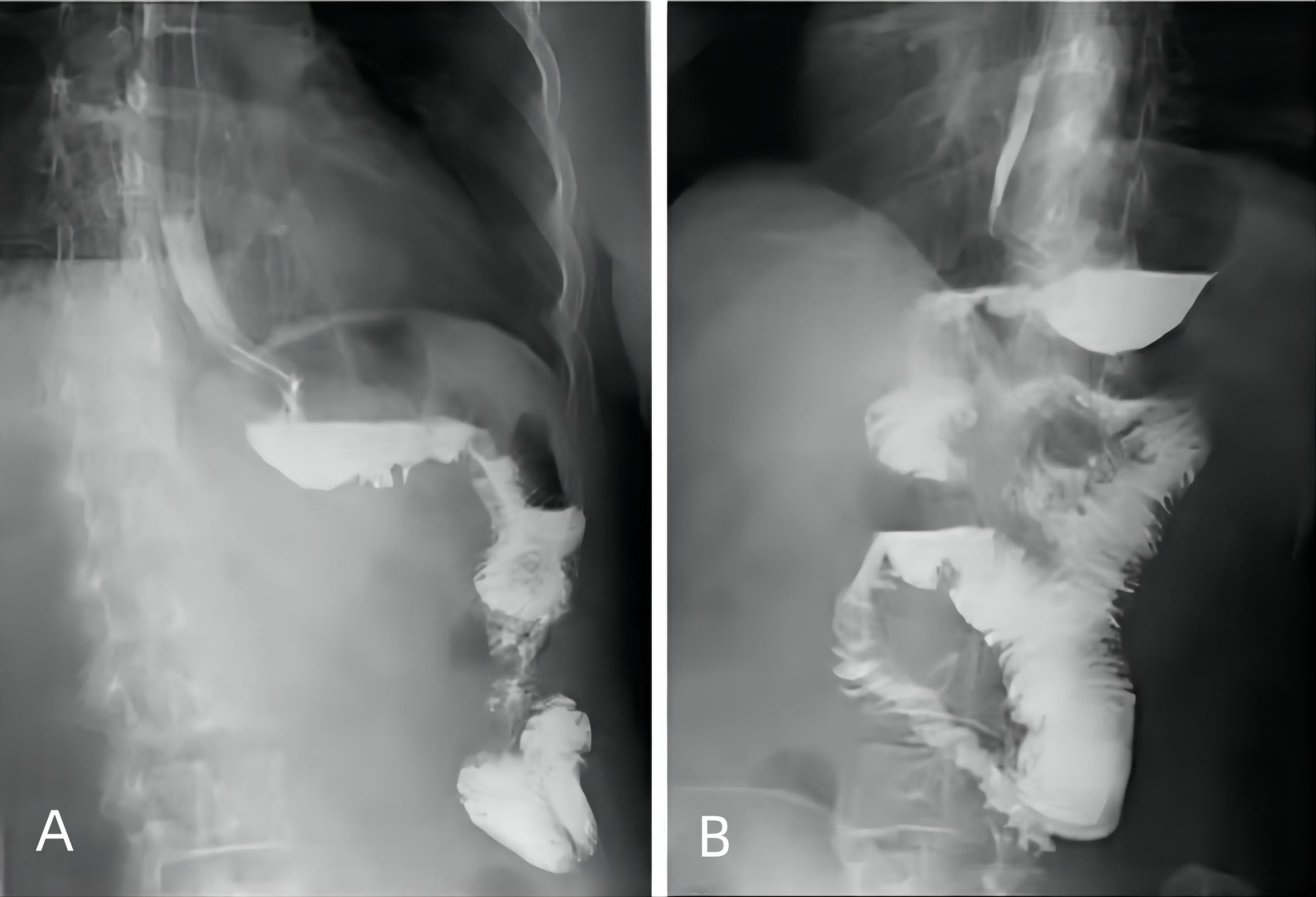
Upper gastrointestinal water-soluble contrast radiography performed 3 days after SPLT-RY in TLDG. (A) Anastomosis of the proximal stomach and distal jejunum. (B) Anastomosis of the small intestine and proximal jejunum. SPLT-RY, self-pulling and latter transected Roux-en-Y; TLDG, total laparoscopic distal gastrectomy.
